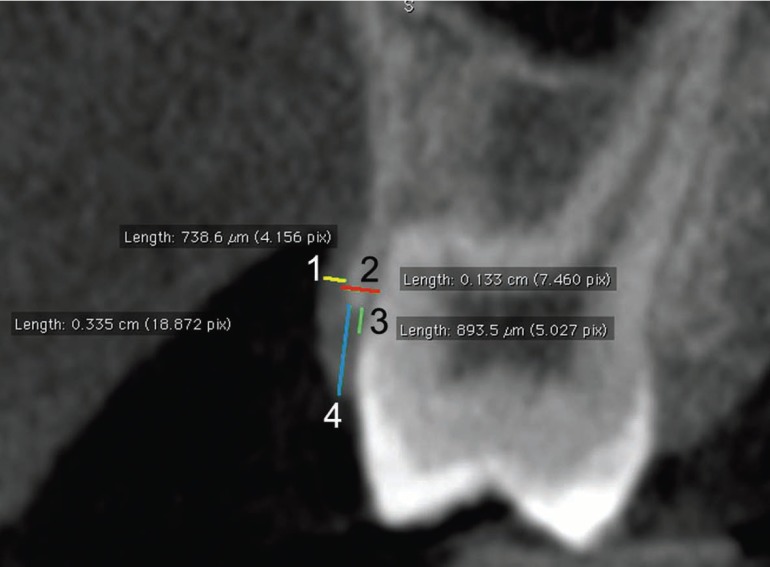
Measurements (n=4) taken through ST-CBCT in each patient to analyze the periodontal effects of rapid maxillary expansion. Legend: Measurements from 1 to 4 correspond, respectively, to the periodontal parameters TAG, BBPT, CJABC and GMABC. Additional description of these parameters is found in  Table 1.
